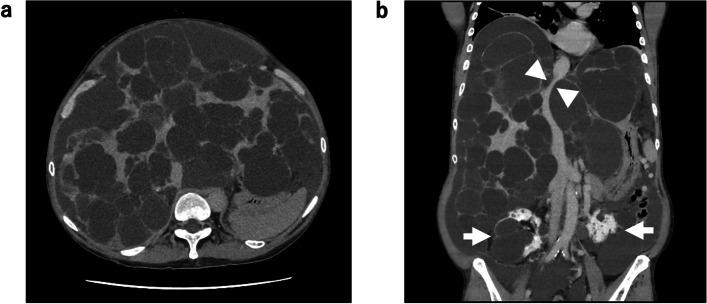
Abdominal contrast-enhanced computed tomography images △Narrowed inferior vena cava (IVC) due to compression by surrounding cysts.  → Polycystic kidneys
